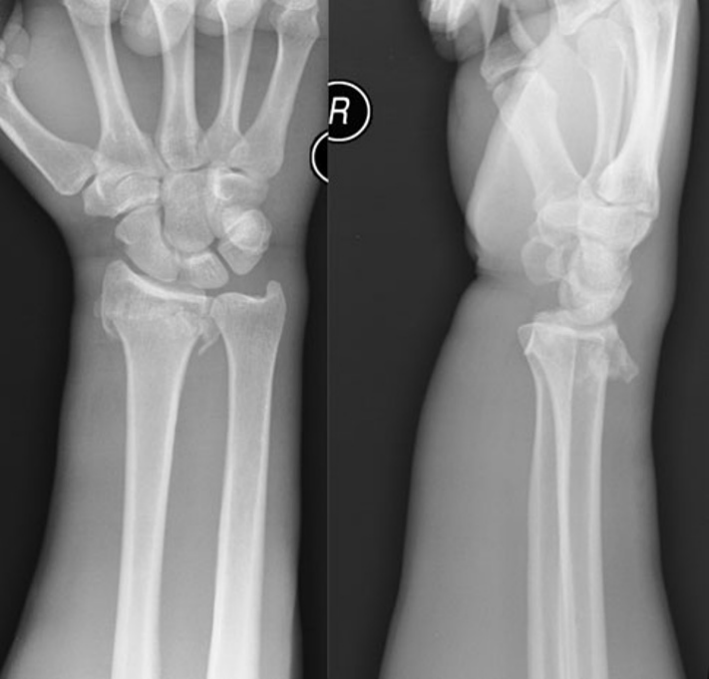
Radiographs after injury, showing the distal radius fracture
